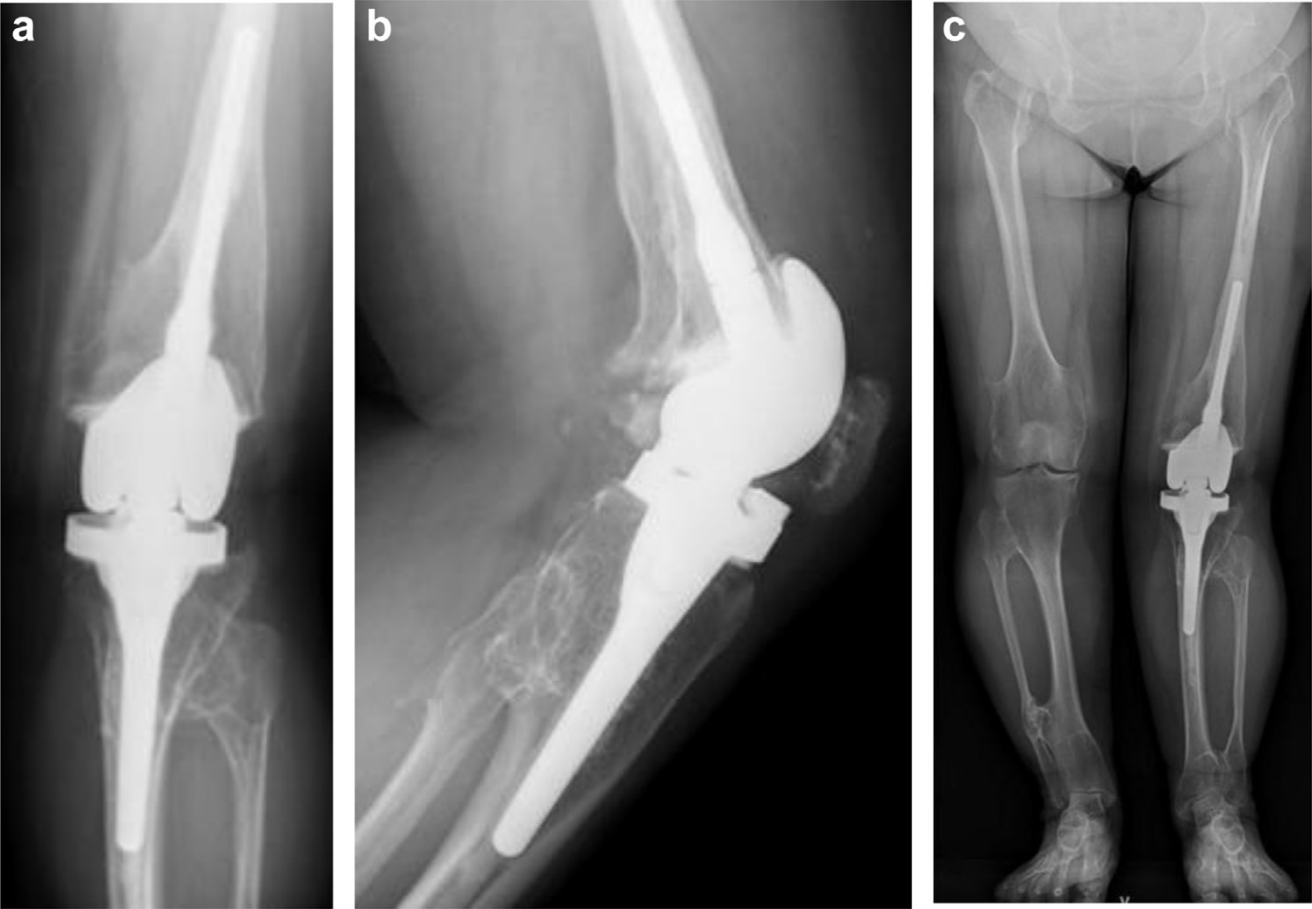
Postoperative (a) anteroposterior, (b) lateral, and (c) full-length radiographs of the left knee at 1-year follow-up. The radiographs demonstrated no evidence of implant loosening, acceptable limb alignment, symmetric pelvic girdles, and 9-degree valgus deformity of the left knee.
